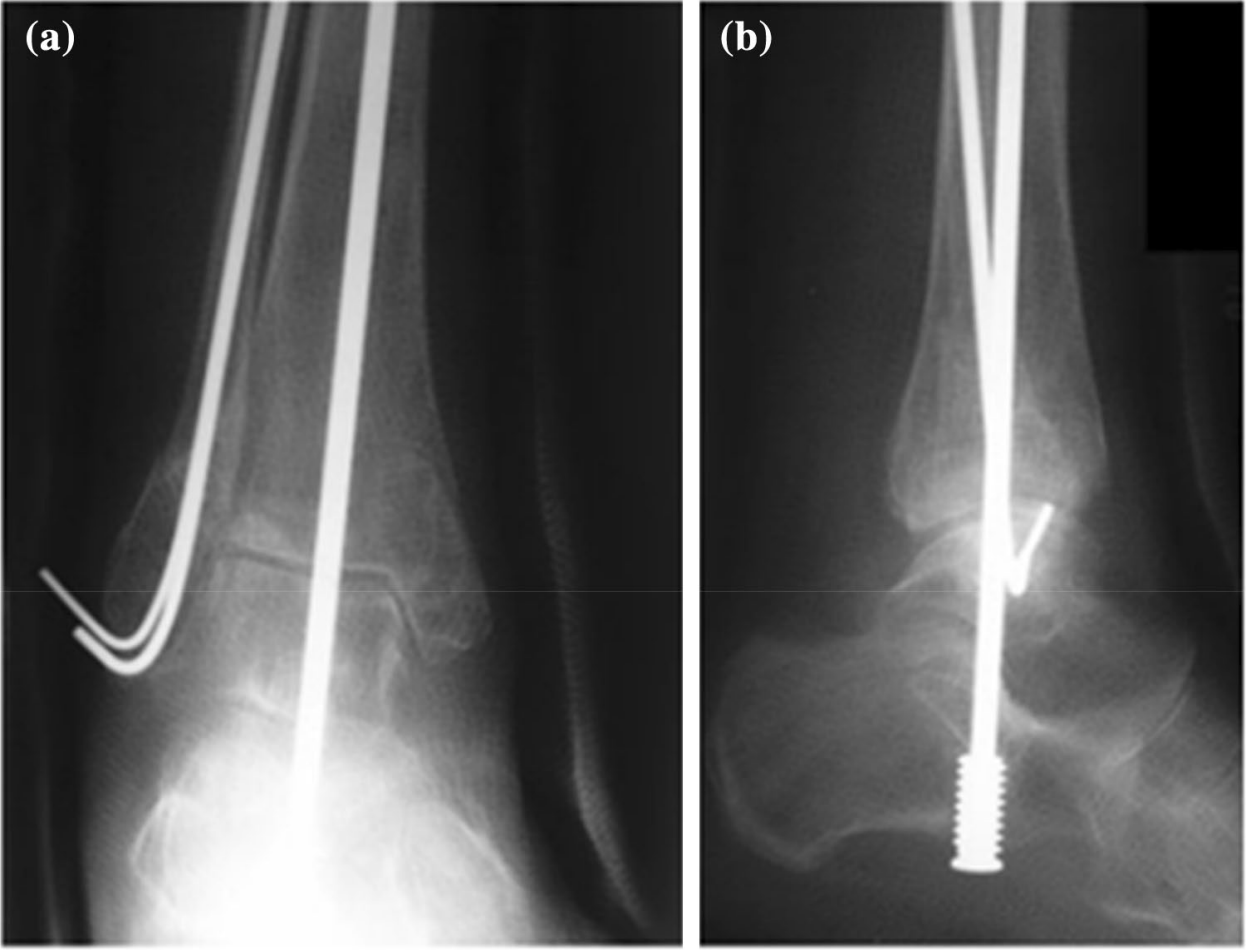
Post-operative a AP X-ray and b Lateral X-ray ankle: Mortice reduction maintained at 6 weeks post-operatively following Gallagher nail insertion. Kirschner wires have been introduced into the lateral malleolus to stabilize the reduction. Source: Obtained from Ref. 23
